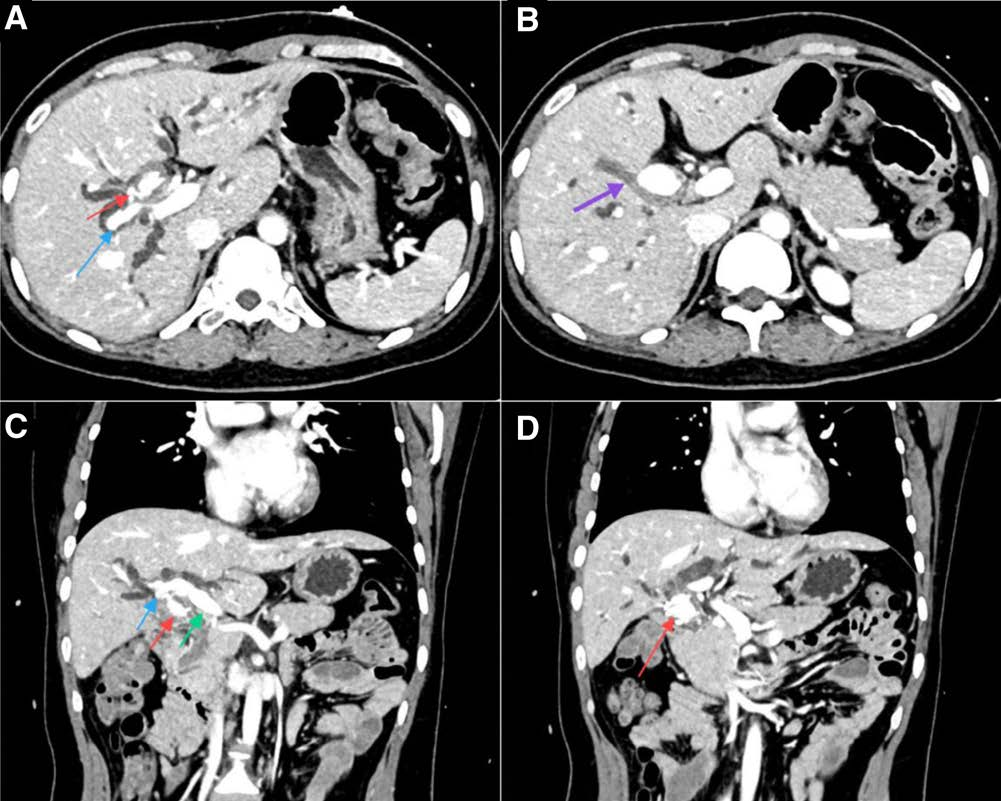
Enhanced CT scan of the chest and abdomen. (A) Axial view showing a high-density lesion (red arrow) at the gallbladder fossa, which is connected to the right hepatic artery (blue arrow). (B) Axial view demonstrating significant dilation of the intrahepatic bile ducts due to compression by the lesion (purple arrow). (C) Coronal view revealing the lesion (red arrow) originating from the right hepatic artery (blue arrow), a branch of the proper hepatic artery (green arrow). (D) The lesion is located at the site of the previously resected gallbladder. CT = computed tomography.
